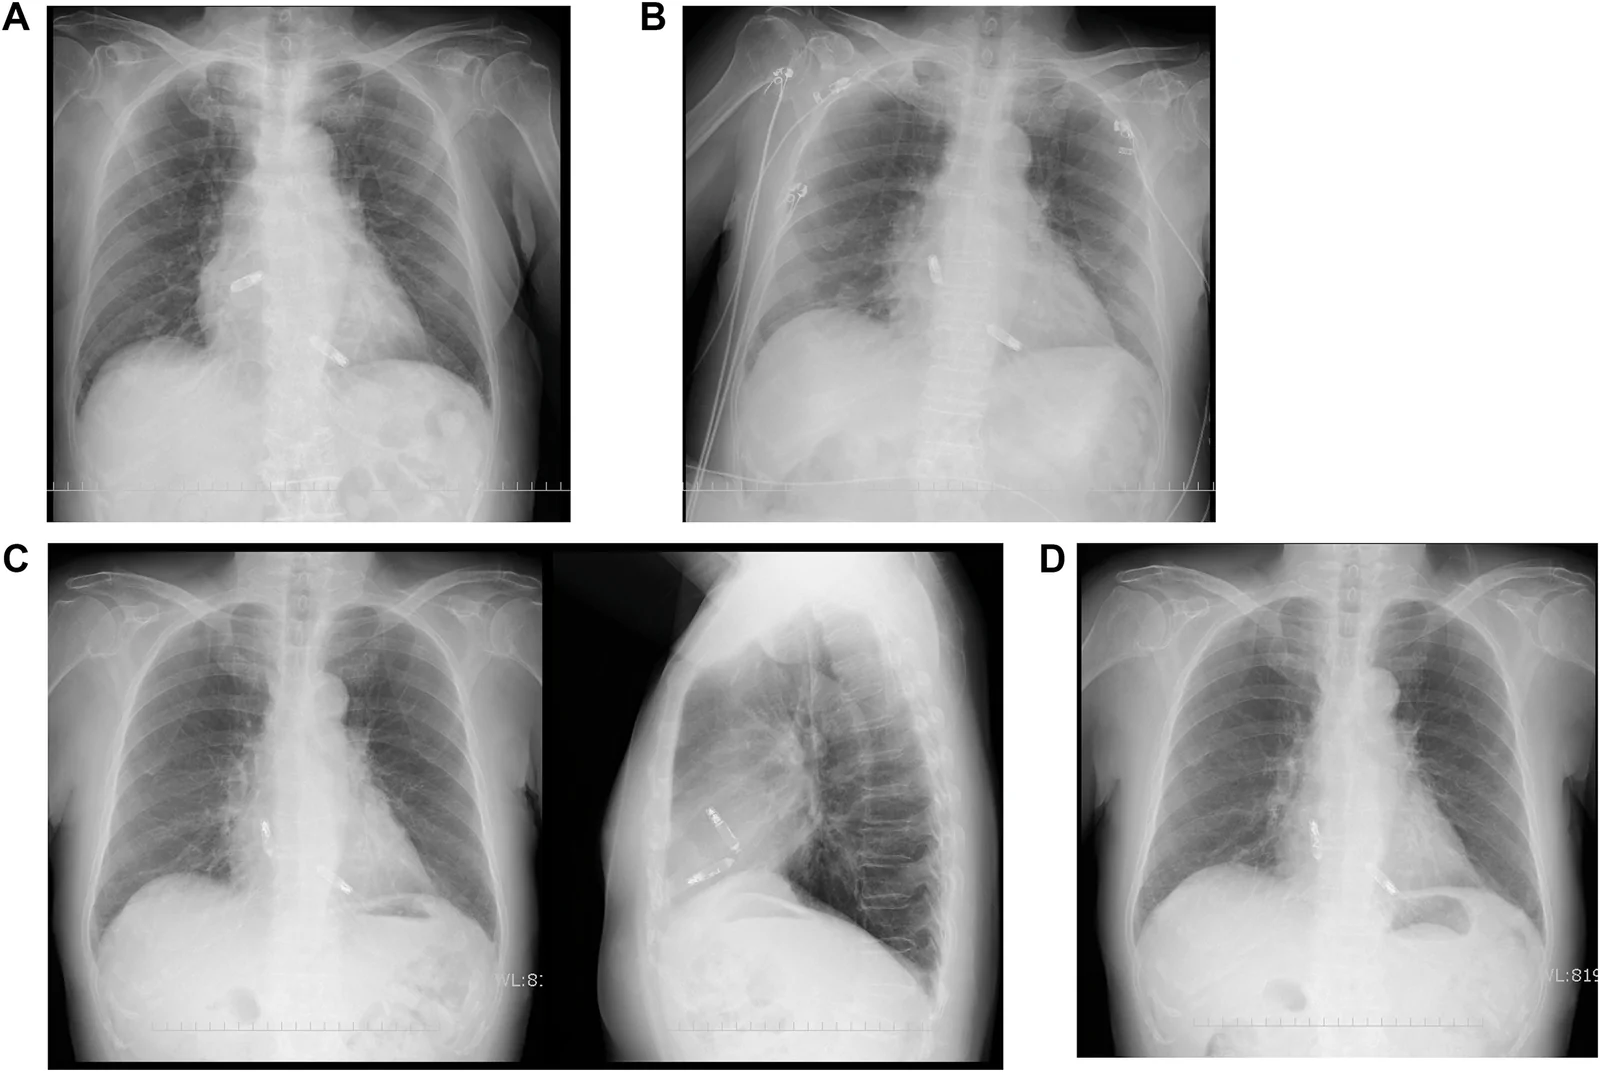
Chest radiographs in case 2. A: Implantation day. B: Postoperative day 1. C: Postoperative day 2. Left panels show anteroposterior views, and right panels show lateral views. D: Postoperative day 4. Subtle device displacement was observed.
